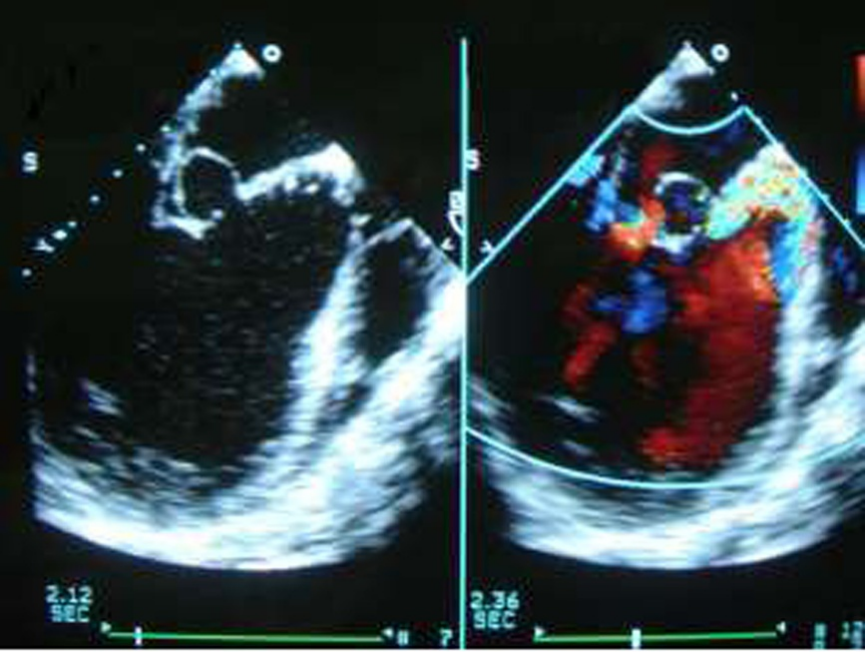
Transesophageal echocardiography during operation revealed a saccular structure in the anterior leaflet that bulged into the left atrium throughout the cardiac cycle.
